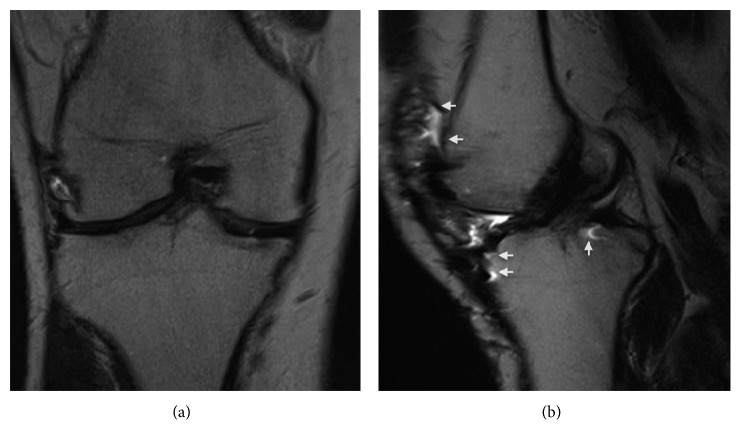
Coronal (a) and sagittal (b) T2-weighted metal reduction magnetic resonance images of the right knee, demonstrating an apparently intact ACL. Note the presence of metallic artifacts (white arrows) throughout the anterior and posterior aspect of the knee on the sagittal image.
